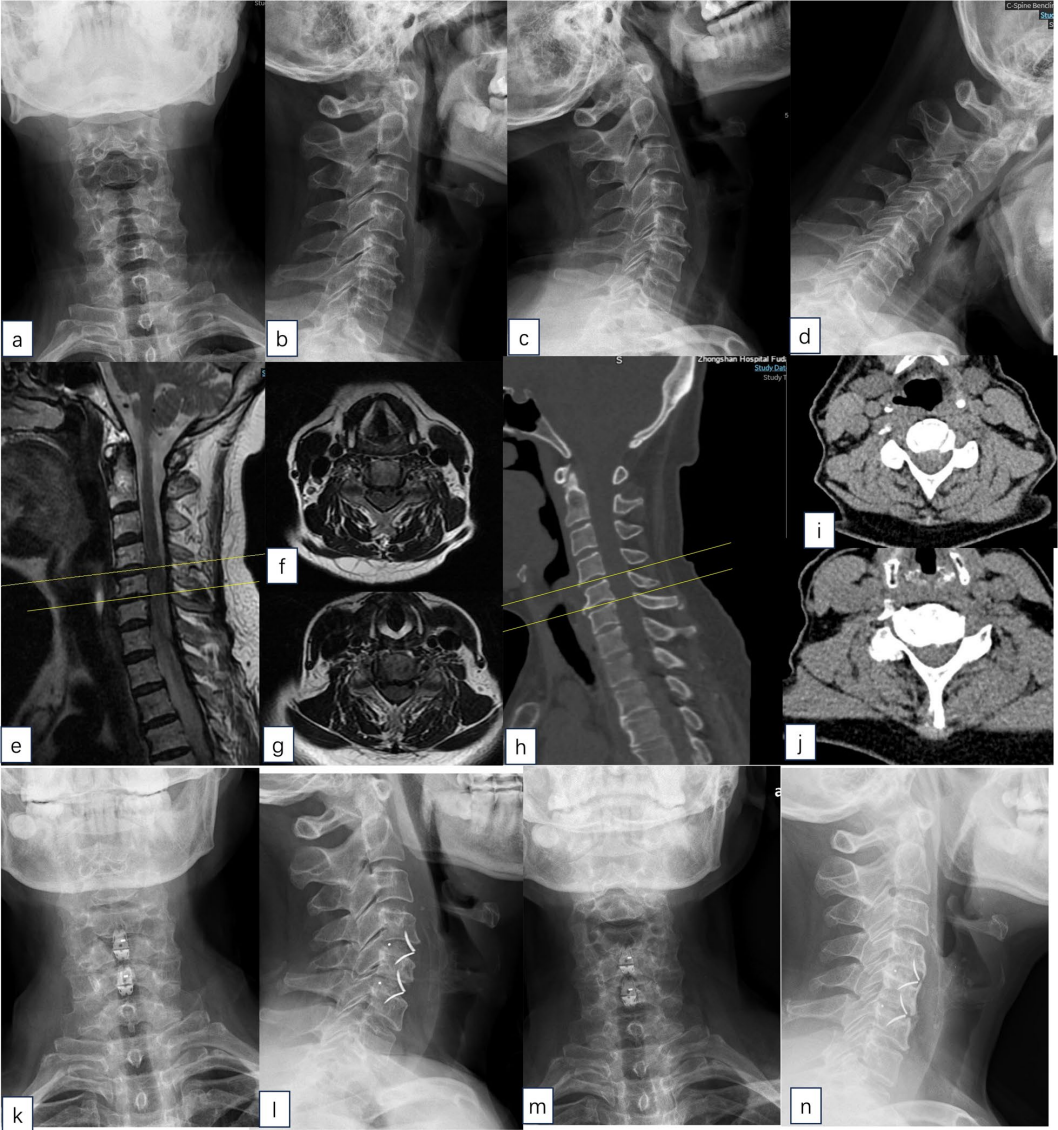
A patient aged 83 years old, who had symptoms of cervical radiculopathy and myelopathy, was treated with two-level ACDF with self-locking standalone cages. a and b Preoperative anteroposterior and lateral X-rays. c and d Flexion–extension cervical spine radiography. e–g Preoperative MRI showed C45 and C56 spinal stenosis and disc herniation. h–j Preoperative CT showed C45 and C56 spinal stenosis and soft disc herniation. k and i Anteroposterior and lateral X-rays at 3 days after the operation. m and n Anteroposterior and lateral X-rays at the final follow-up
